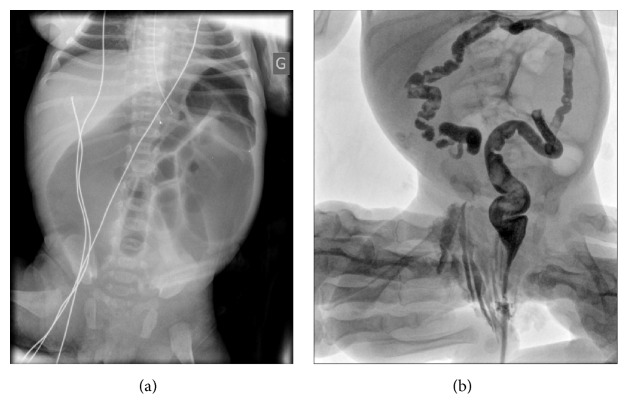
Postnatal plain abdominal X-ray showing a voluminous dilated bowel loop (a). Water-soluble contrast enema with opacification of a few centimeters of the last ileal loop (b).
